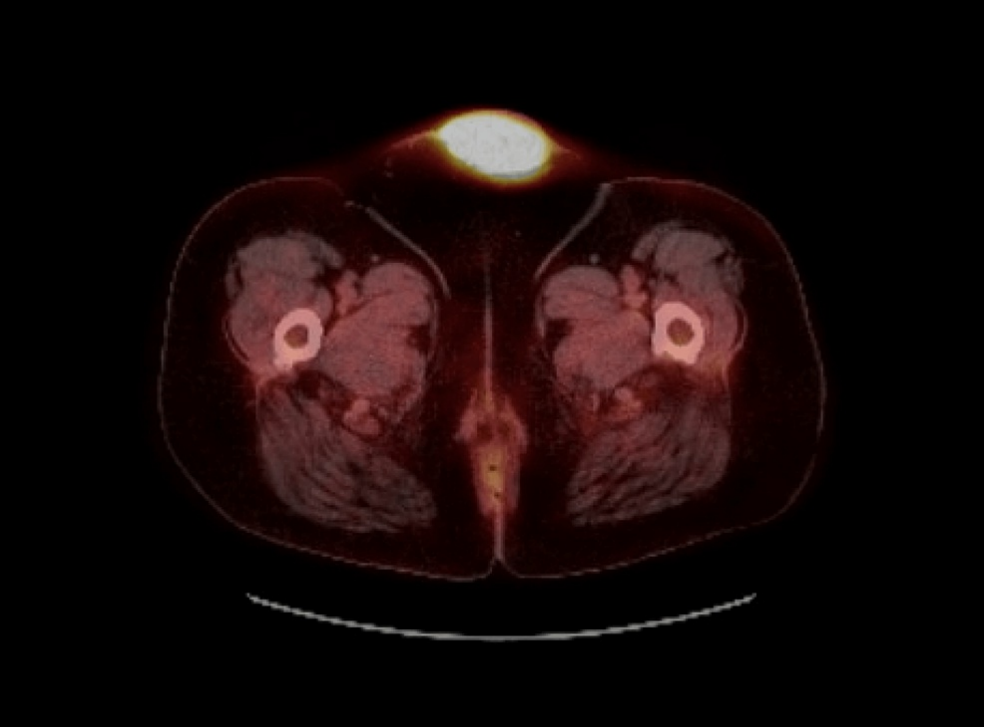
Transverse view of the CT-PET.
